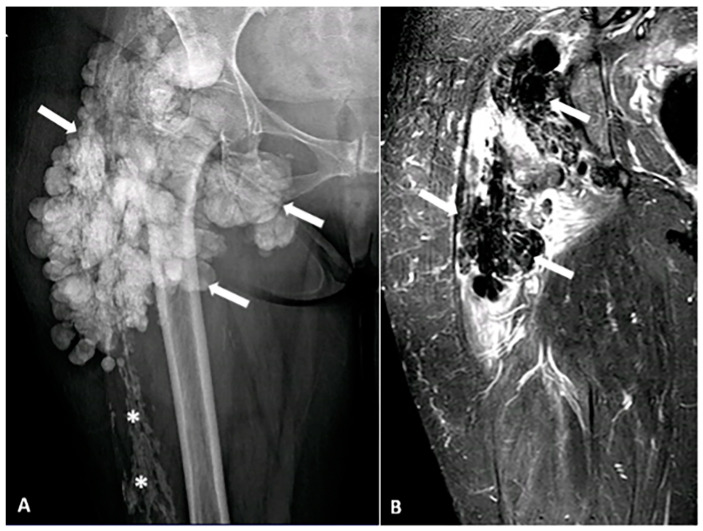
A 52-year-old female with a firm, palpable mass in the right hip. She also has sclerodactyly, dysphagia, Raynaud’s phenomenon, and polyarthralgia. (A) Anteroposterior (AP) radiograph of the femur shows clustered, lobulated calcifications (arrows) consistent with pseudotumoral calcinosis, which can be associated with connective tissue diseases. Sheet-like calcifications (star) indicating calcinosis cutis, commonly associated with systemic sclerosis. (B) Coronal MR STIR image demonstrates confluent areas of signal voids, corresponding to foci of calcification. The image was obtained from the University of Washington Medical Imaging database with the required permissions.
